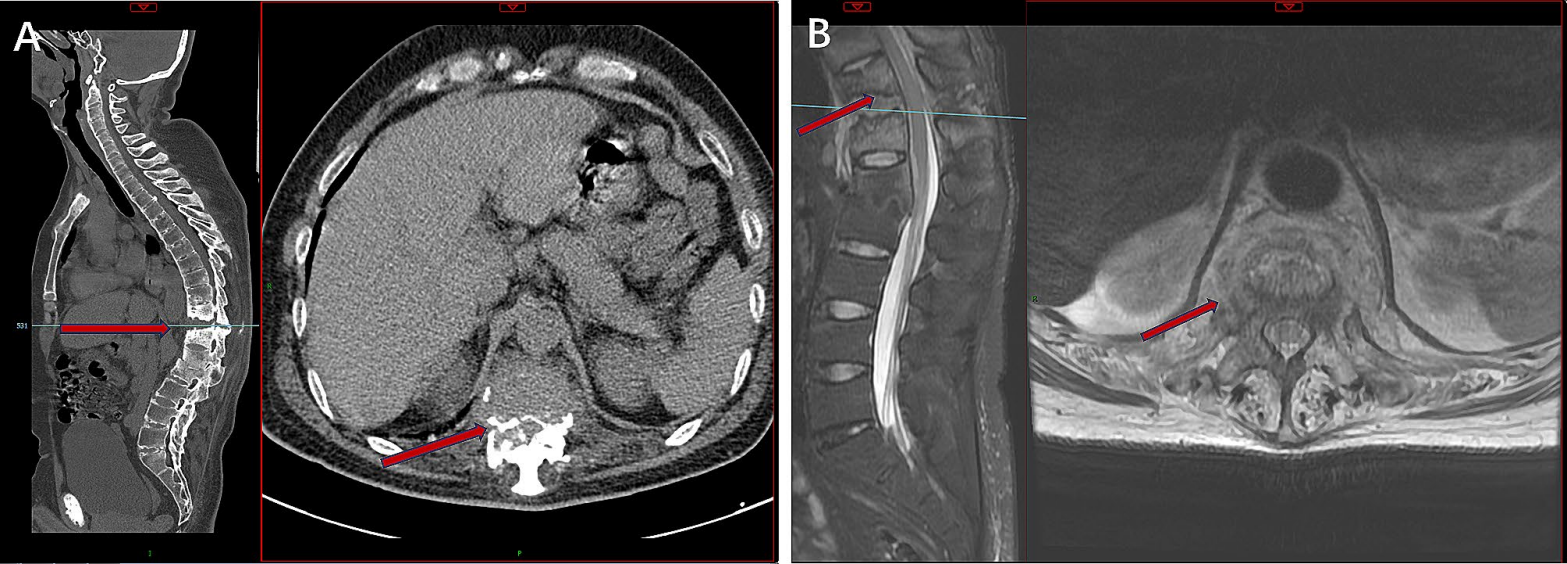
A: The sagittal and axial computed tomography scans indicate significant thoracic kyphosis and osteolysis in the T11/12 intervertebral space (arrow), with irregular sclerotic zone surrounding it and lesions extending to the posterior elements. B: Magnetic resonance imaging shows erosion of the intervertebral disc and adjacent endplates between T11 and T12 (arrow)
